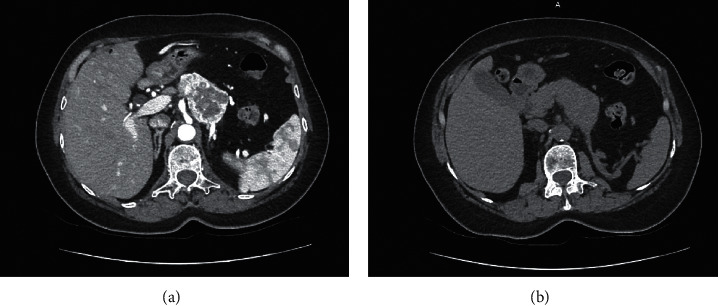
Computed tomography images of a tissular corporeal pancreatic mass, enhanced in periphery (a) with a central necrosis, invading the splenic vein (a, b).
